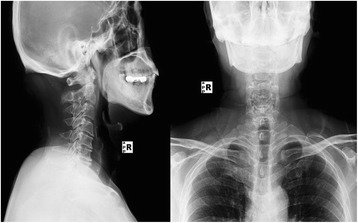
Preoperative x-ray of a 62-year-old female patient with cervical myelopathy
